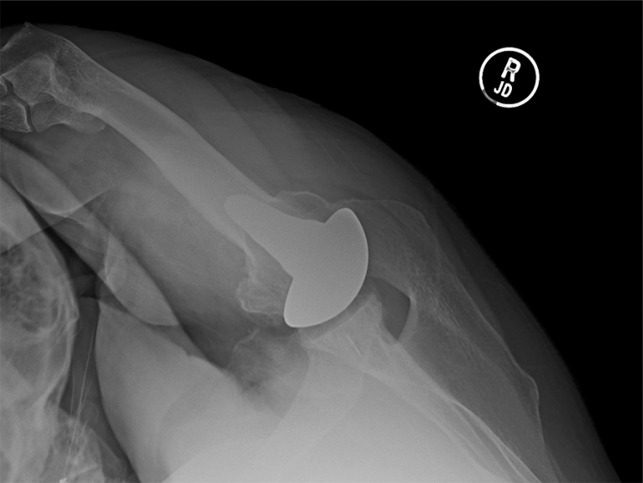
Radiograph showing the example of a healed, union of a lesser tuberosity osteotomy.
